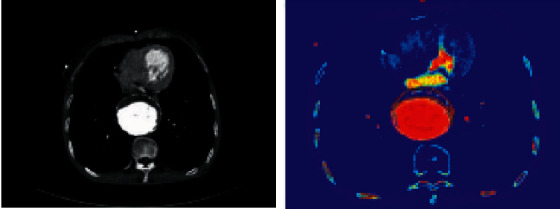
The CT images of aortic valves and the results of target region segmentation in CT images by the algorithm.
